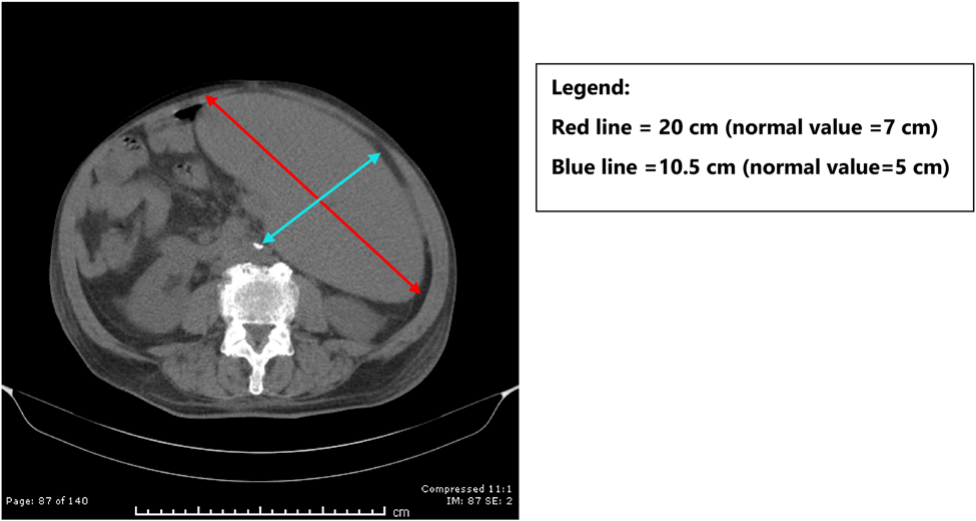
CT scan showing massive splenomegaly.
